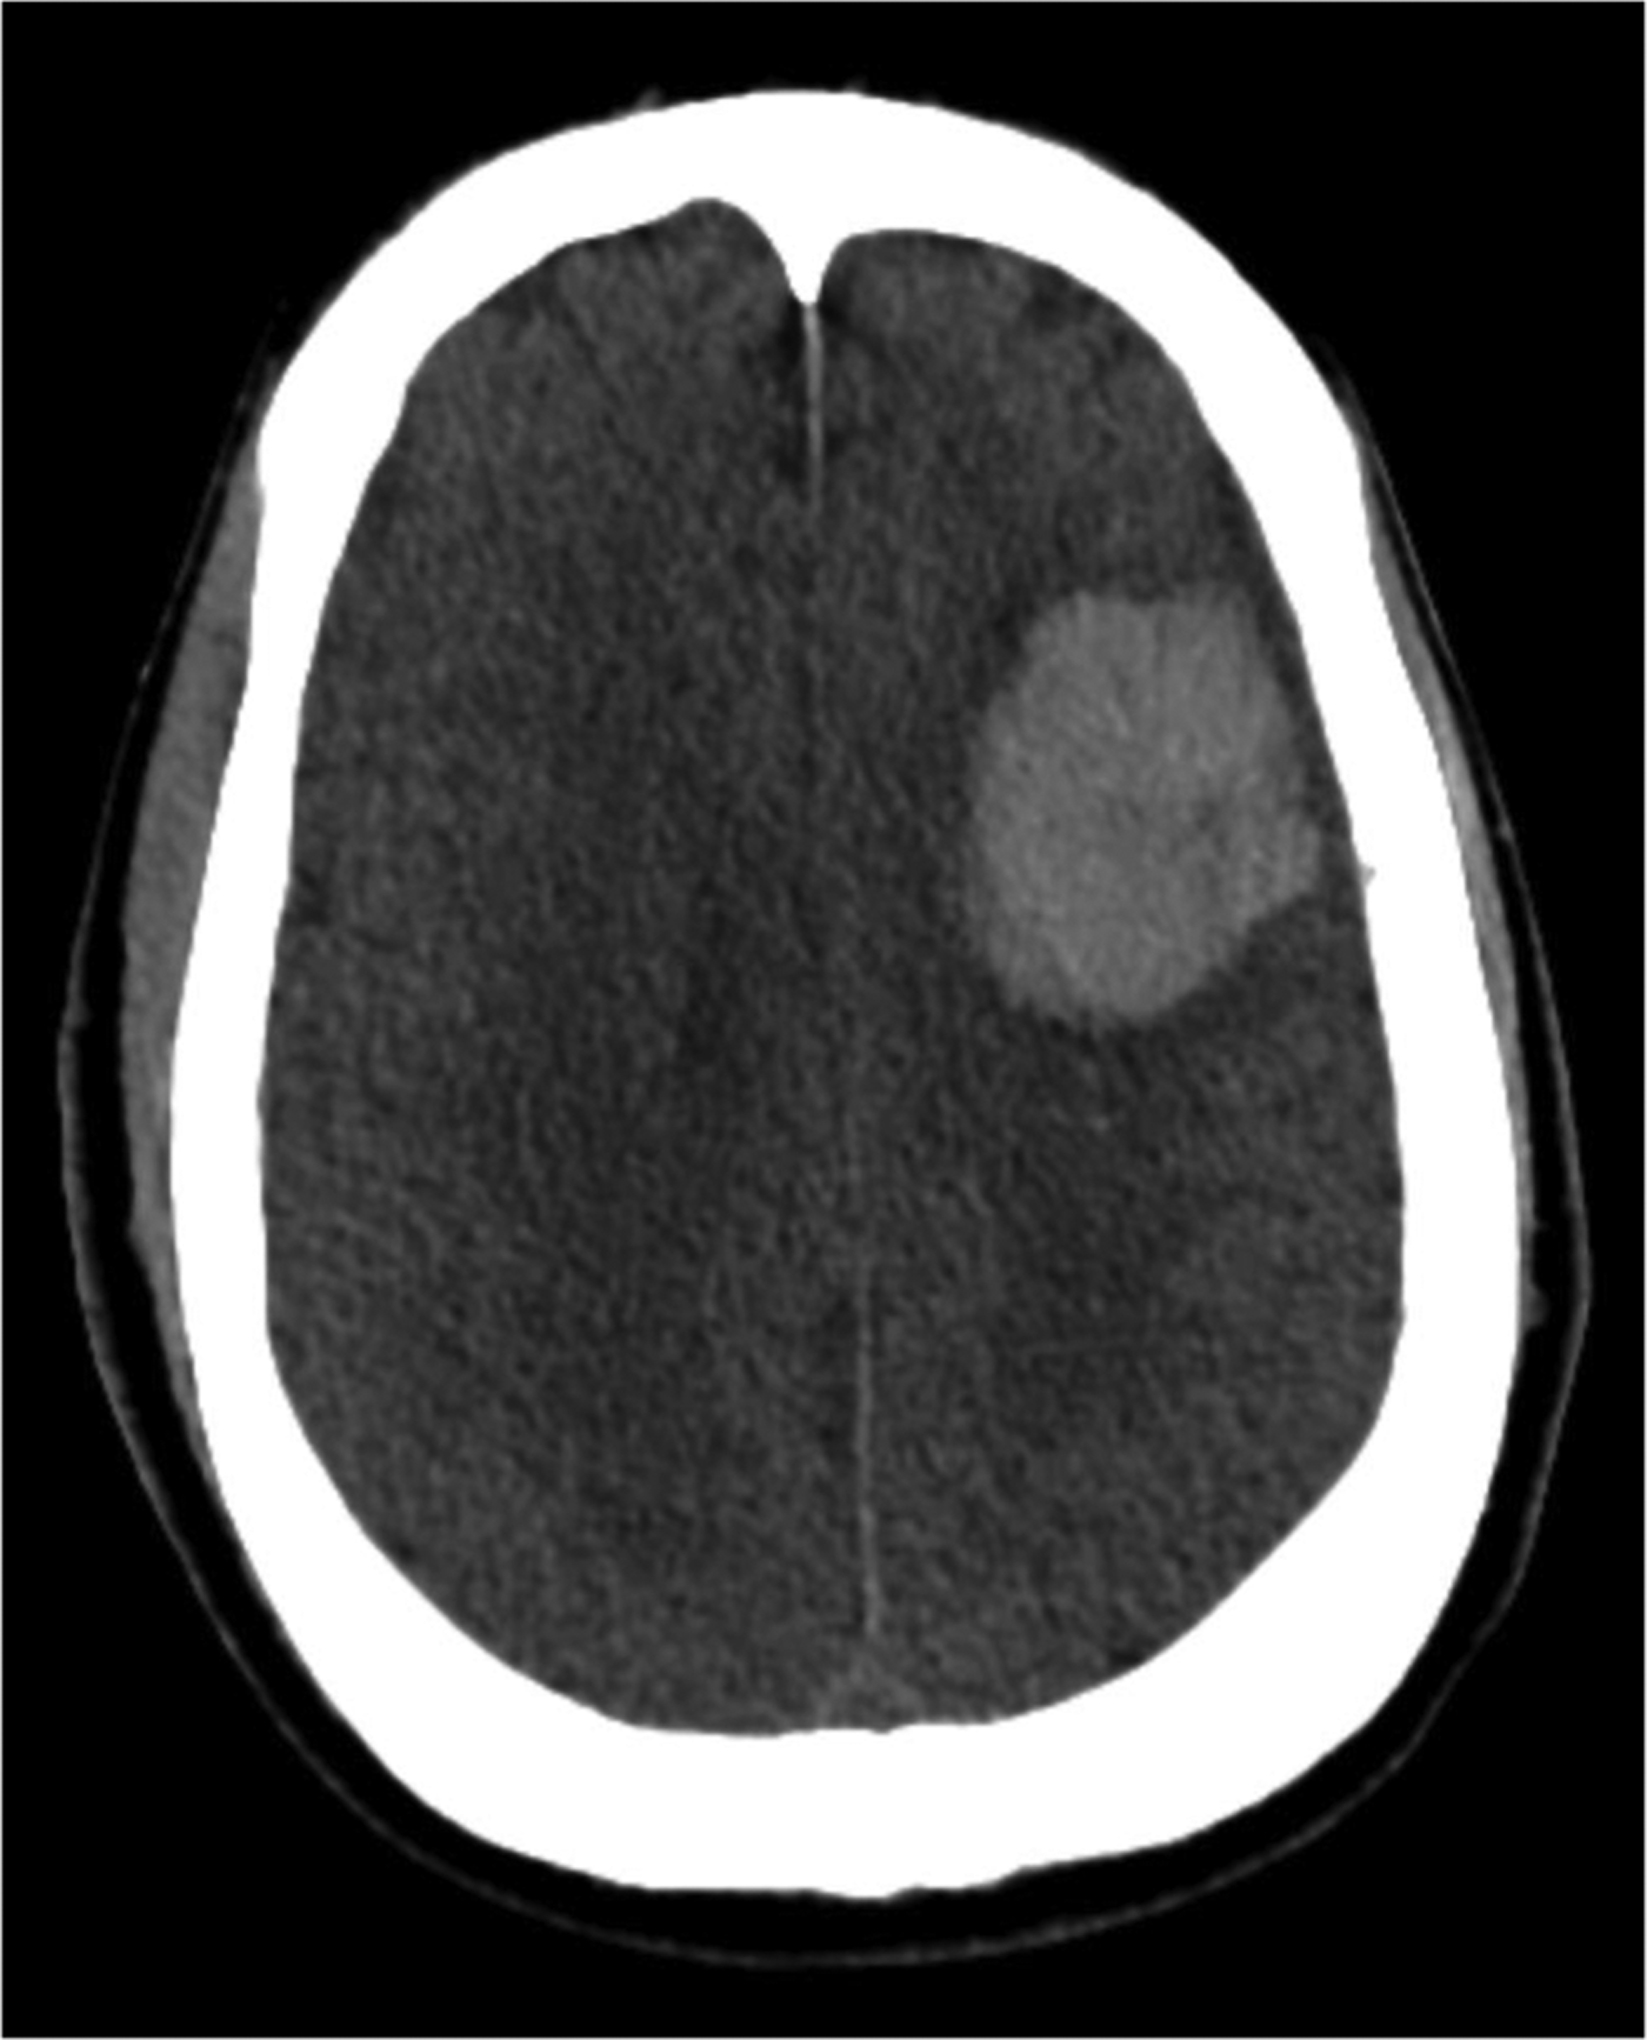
Axial head CT shows a 4 cm left frontal lobe intraparenchymal hemorrhage.
